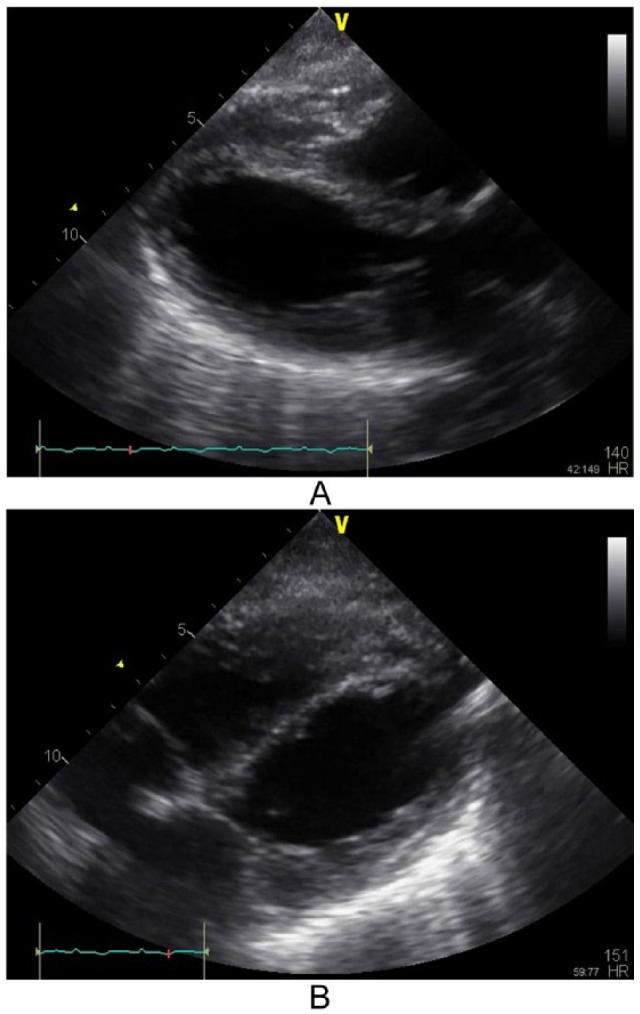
Transthoracic echocardiogram images showing left ventricle during peak systole demonstrating apical akinesis typical of stress cardiomyopathy: (A) parasternal long axis; (B) subcostal 4-chamber view.
